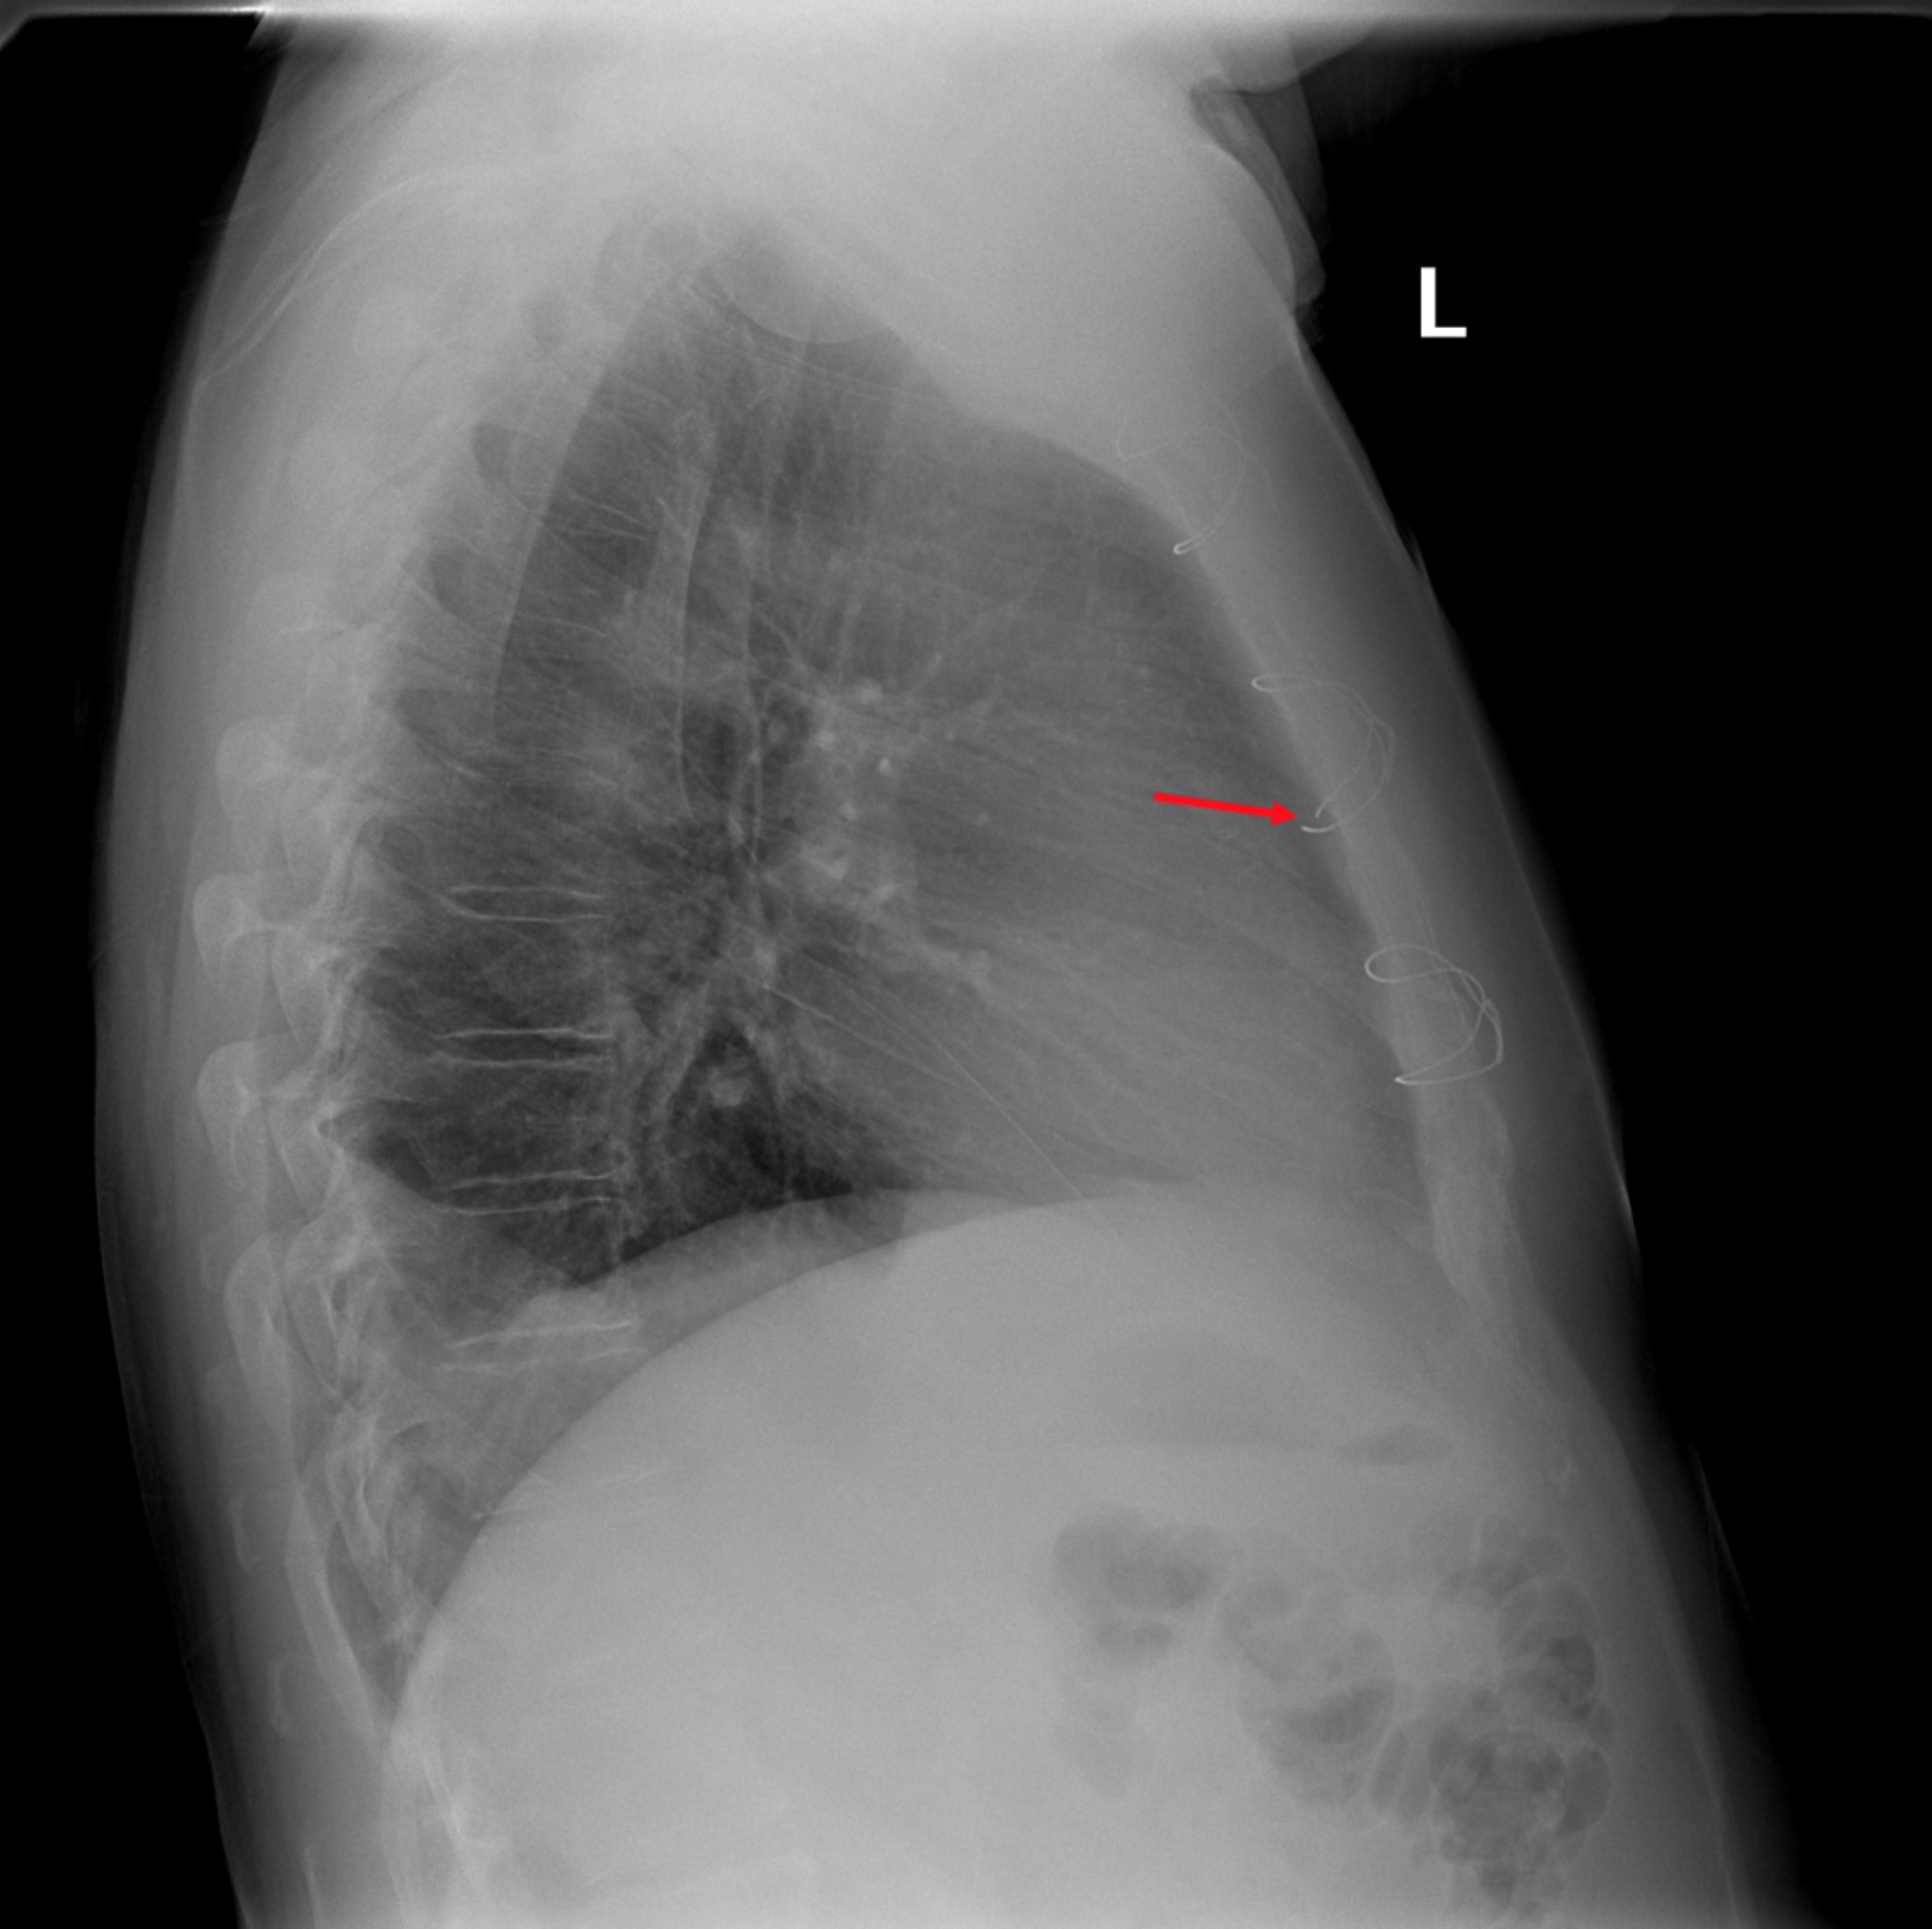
Lateral chest X-ray from five months prior demonstrating a fractured sternal wire located at the posterior sternal table (arrow)
